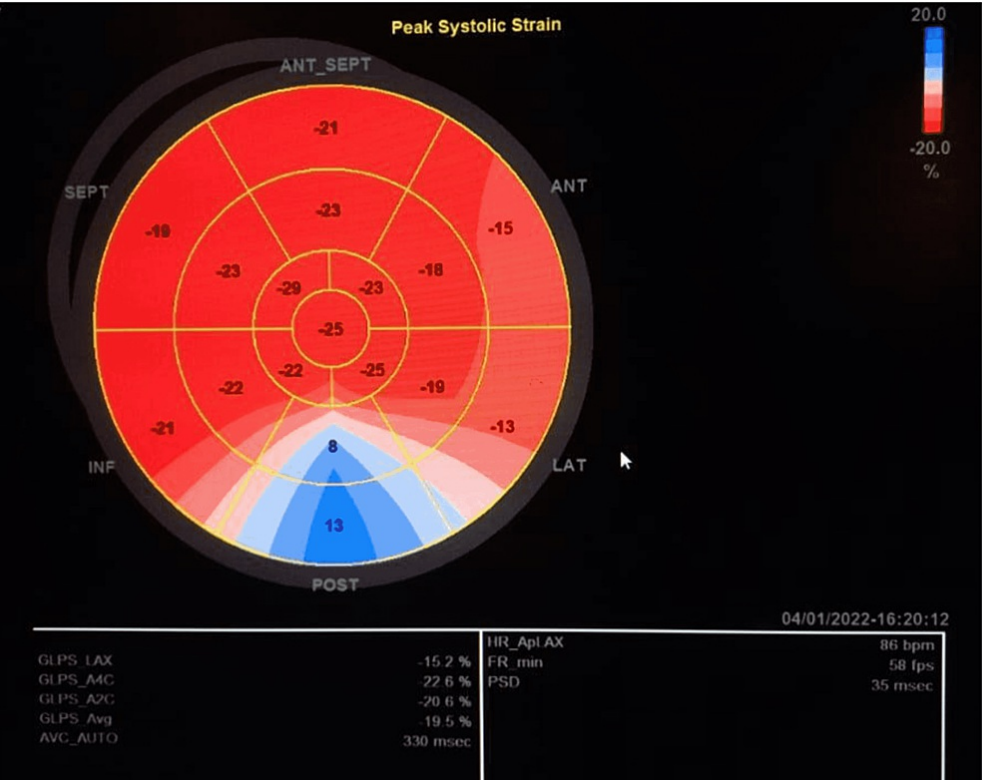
Echocardiography showing decrease in longitudinal strain mainly in posterior segment.
